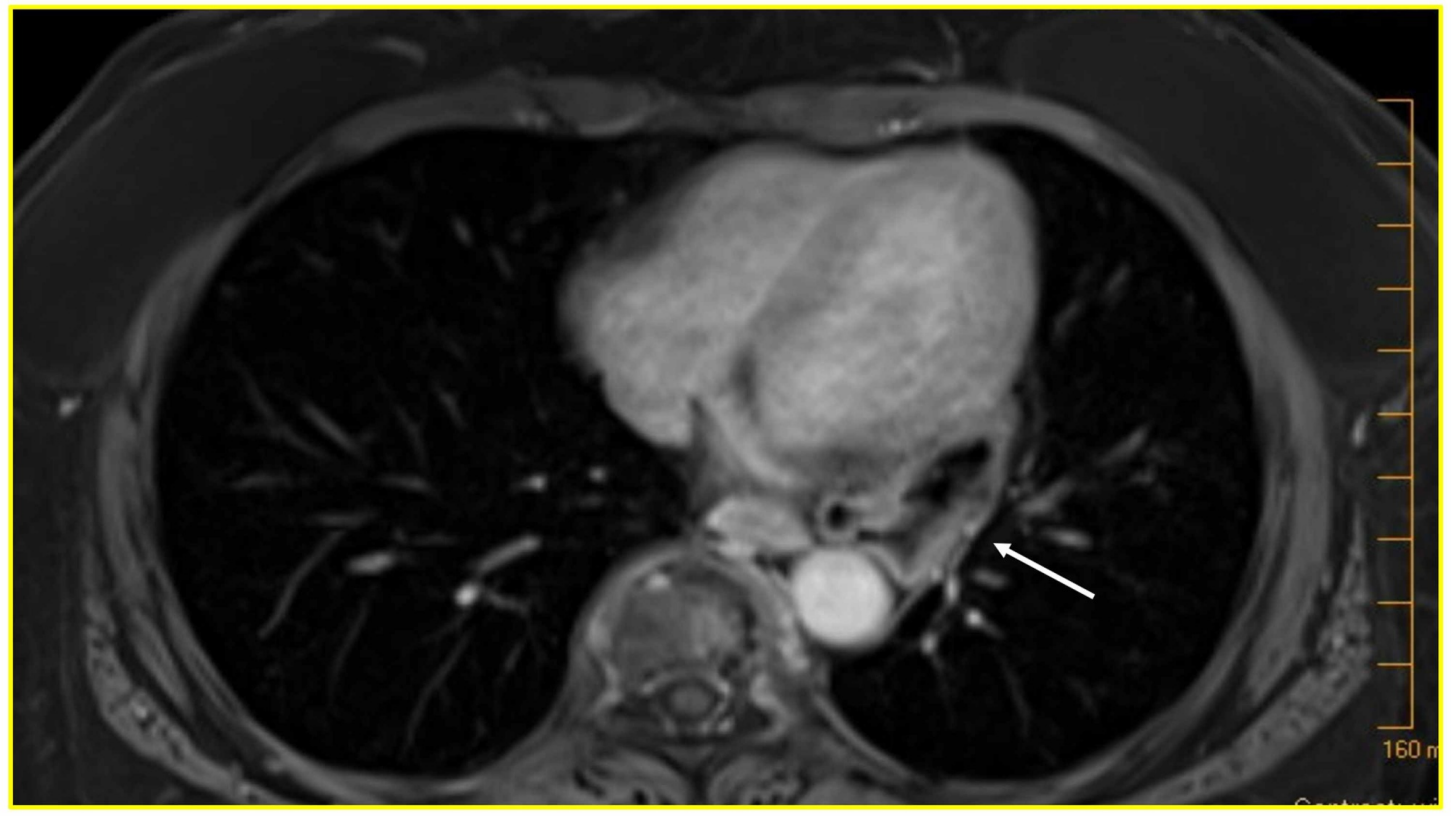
Gastric pouch herniation 12 years post Roux-en-Y bypass surgery T1-weighted axial magnetic resonance image abdomen and pelvis with gadolinium contrast demonstrating the herniated gastric pouch (white arrow, herniated gastric pouch).
